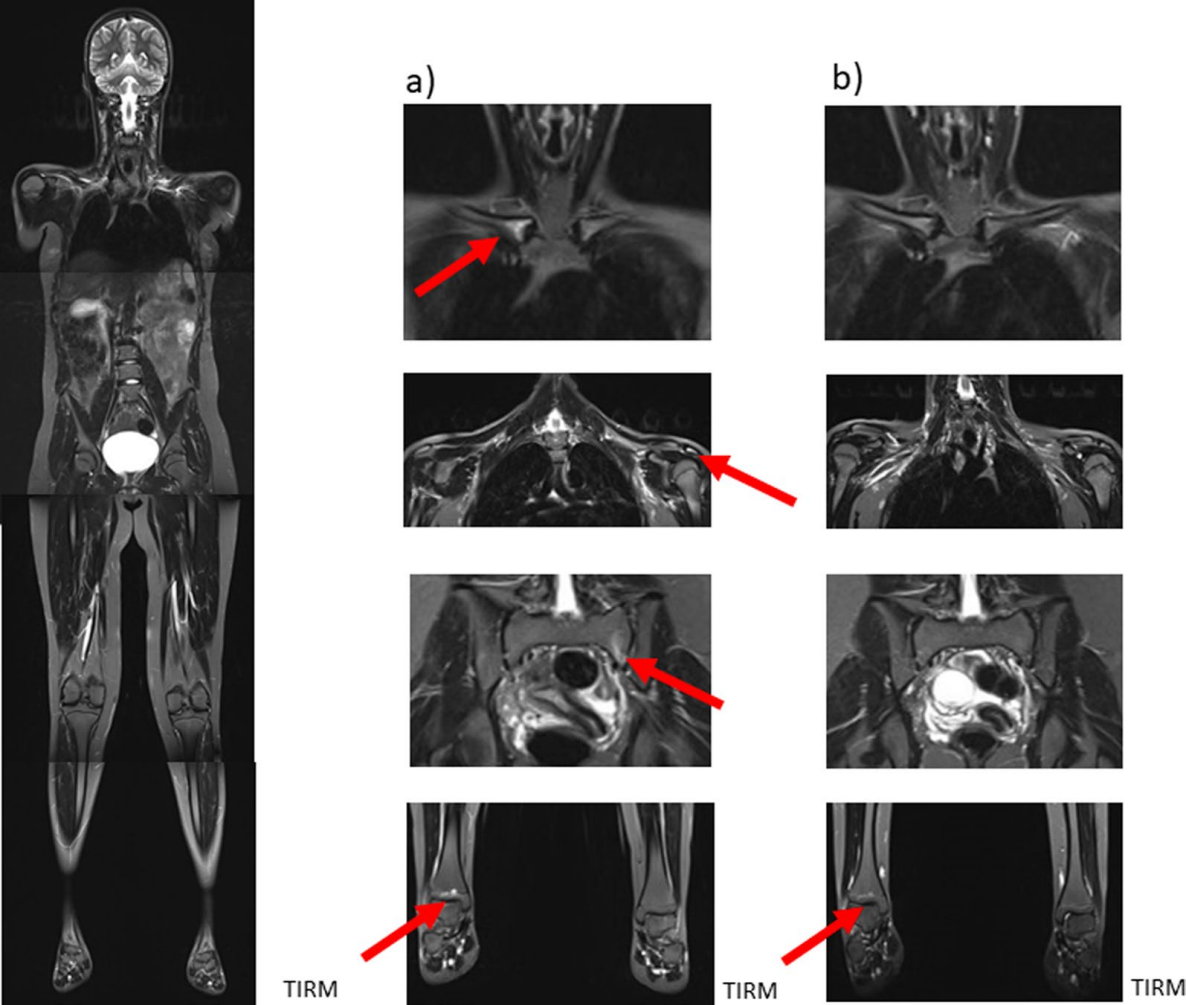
A 12-year-old girl, multifocal CNO. Whole-body MRI (left column) with (foci in the right clavicle, left acromion, left pars lateralis ossis sacri and distal right tibia (enlargements in the middle column). After therapy with naproxen and pamidronate, subtotal regression of the foci (right column)
